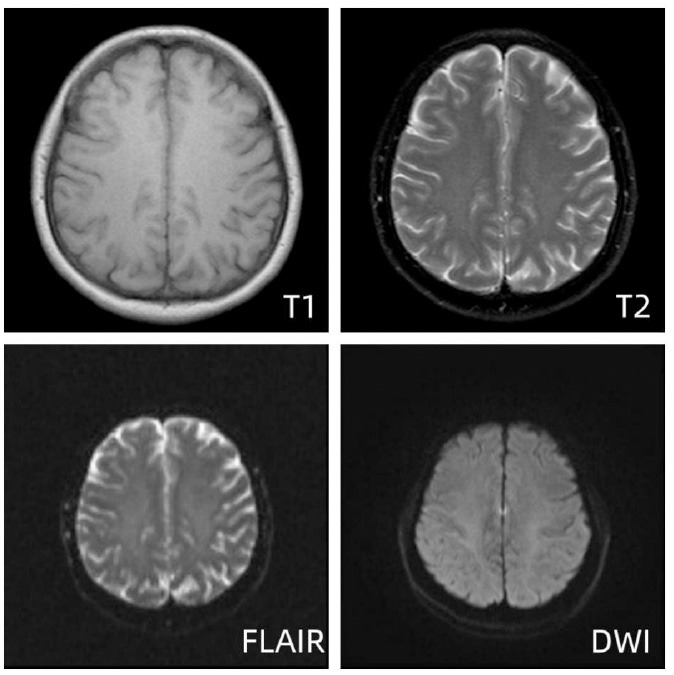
Follow-up MRI of the patient 7 months after discharge from the hospital MRI showed no significant abnormal signals within the brain parenchyma.
